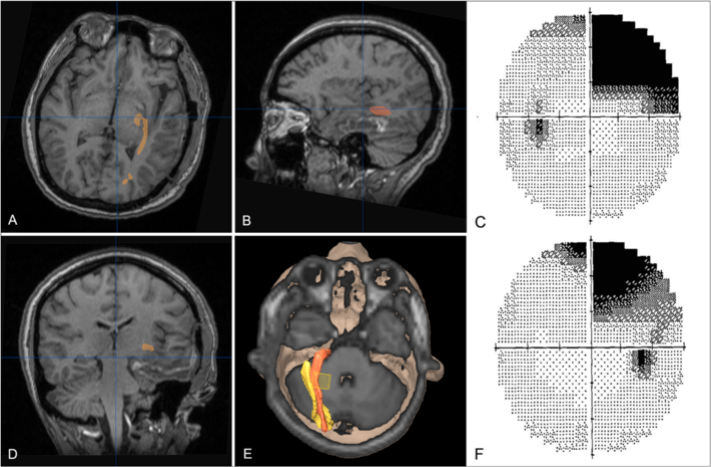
Examination 1 week after surgery. The same patient as in Figs. 1 and 2. Postoperative MRI showed that the dorsal bundle of the optic radiation was well preserved, while anterior tip of Meyer’s loop was damaged (a, b, d, e; *brown*, dorsal bundle; *yellow*, Meyer’s loop). The patient developed right superior quadrantanopia of both eyes (c, f)
